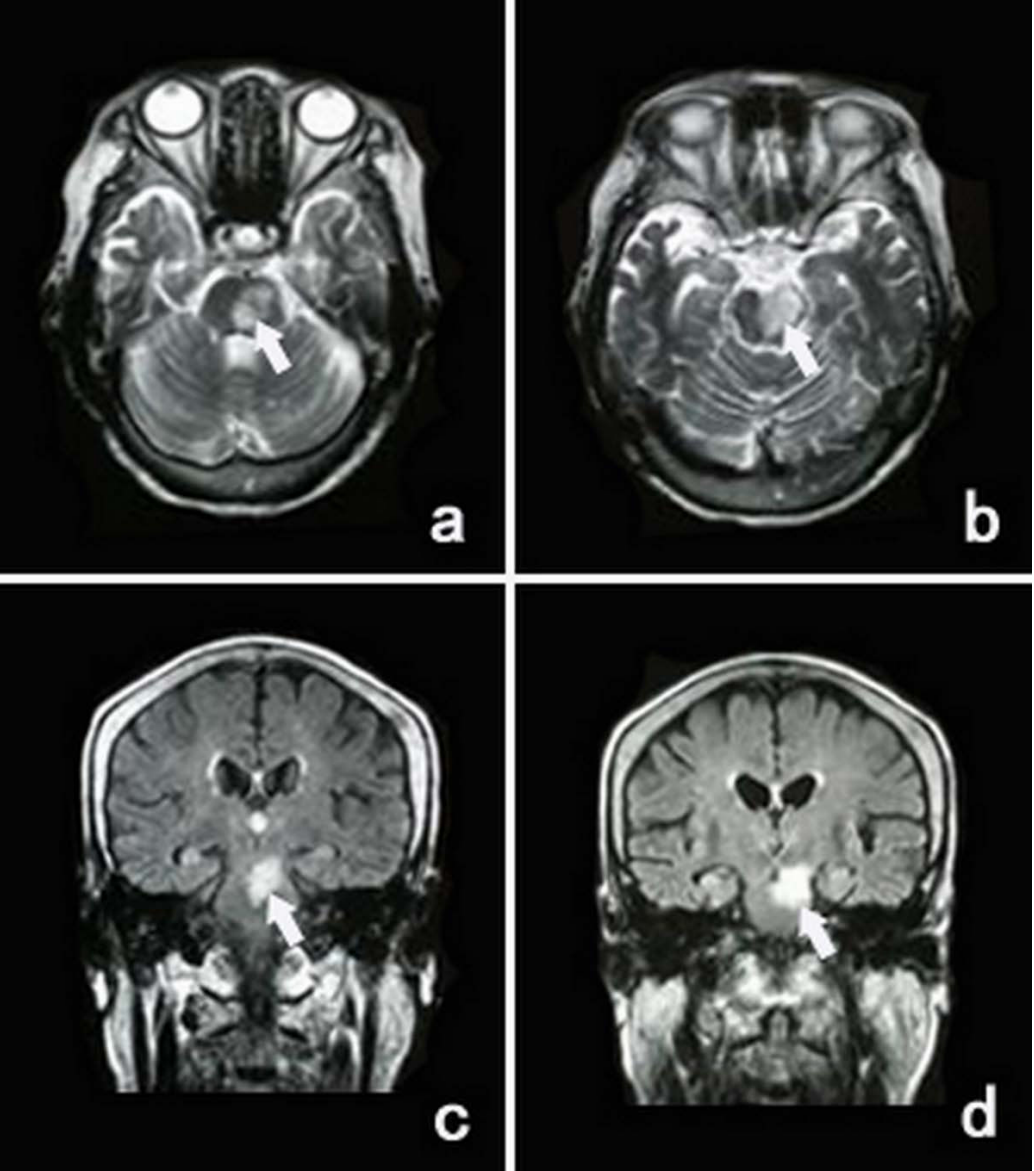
Transversal T_2 _weighted imagings (a, b) and coronal FLAIR sequence (c, d) of MR show the infarction in the pons extending to the midbrain in case 1 (arrows show).
